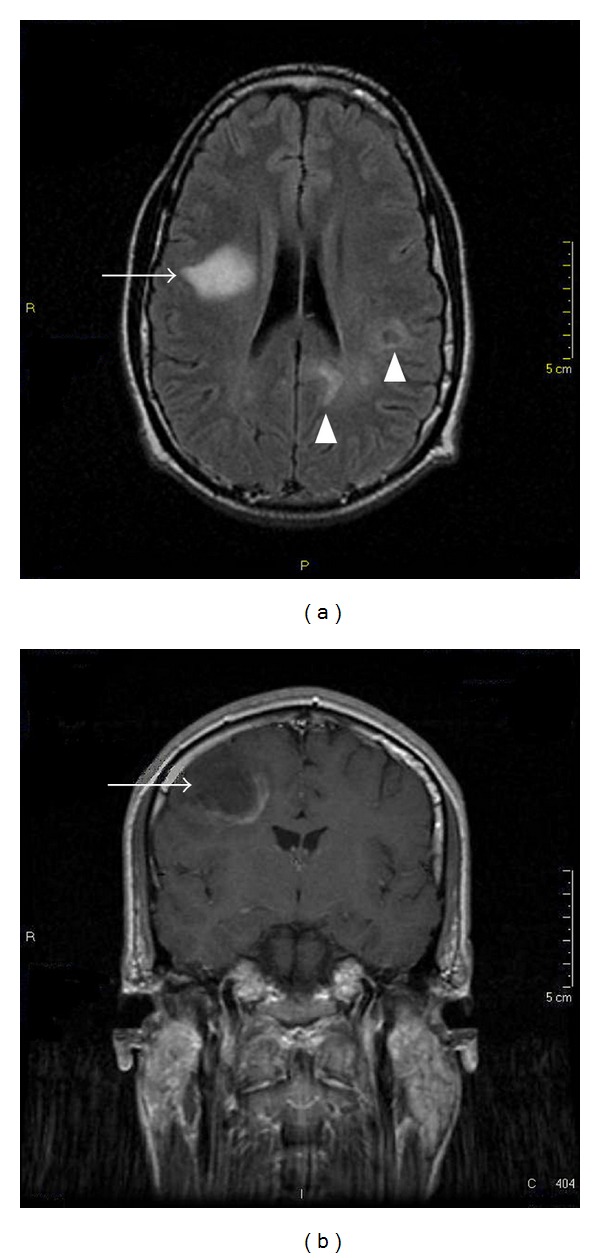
MRI scan after initial therapy. After two cycles of HD-MTX-based chemotherapy, the axial FLAIR image (a) shows good response of left brain masses (arrowheads) but progression of right frontal one (arrow) with contrast reinforcement on the T1-weigthed sequence with gadolinium (b).
